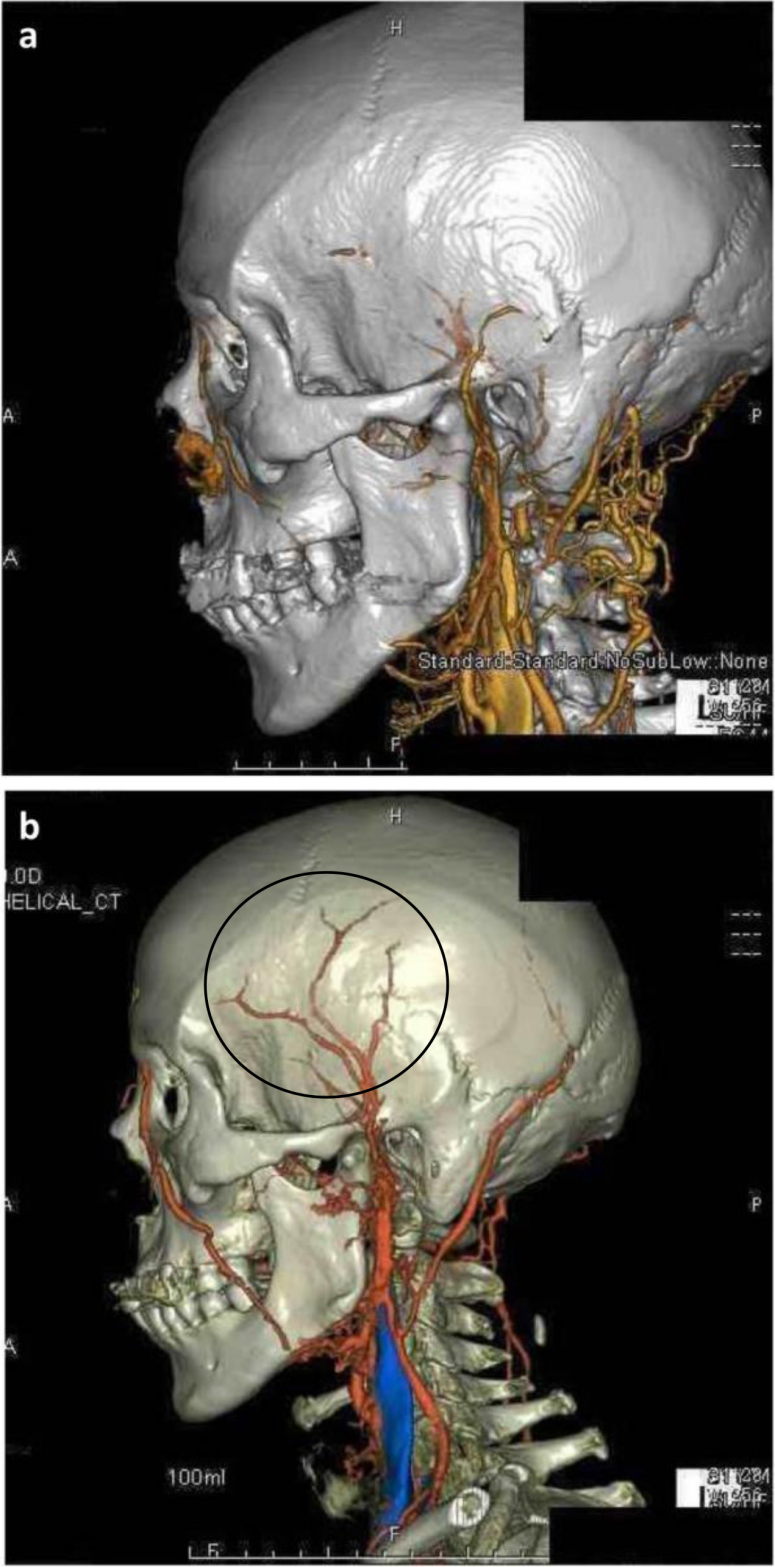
(a) 3D CTA prior to therapeutic immunosuppressive therapy for GCA. (b). 3D CTA after therapeutic immunosuppressive therapy with glucocorticoids demonstrating opening of the temporal artery (circle). 3D, three-dimensional; CTA, CT angiography; GCA, giant cell arteritis.
